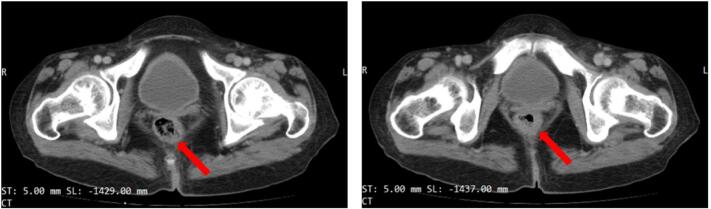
CT scan showing thickened rectal wall.
